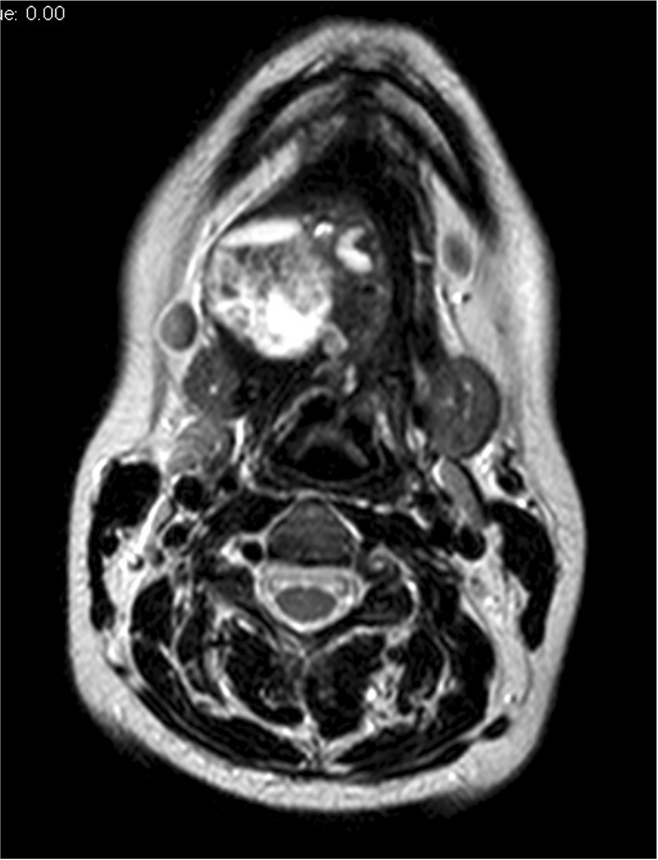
Axial T2-weighted turbo spin echo unenhanced sequence without fat saturation shows a 4.5 × 4.0 × 3.7-cm hyperintense, rough oval formation suspected to contain mucus.
